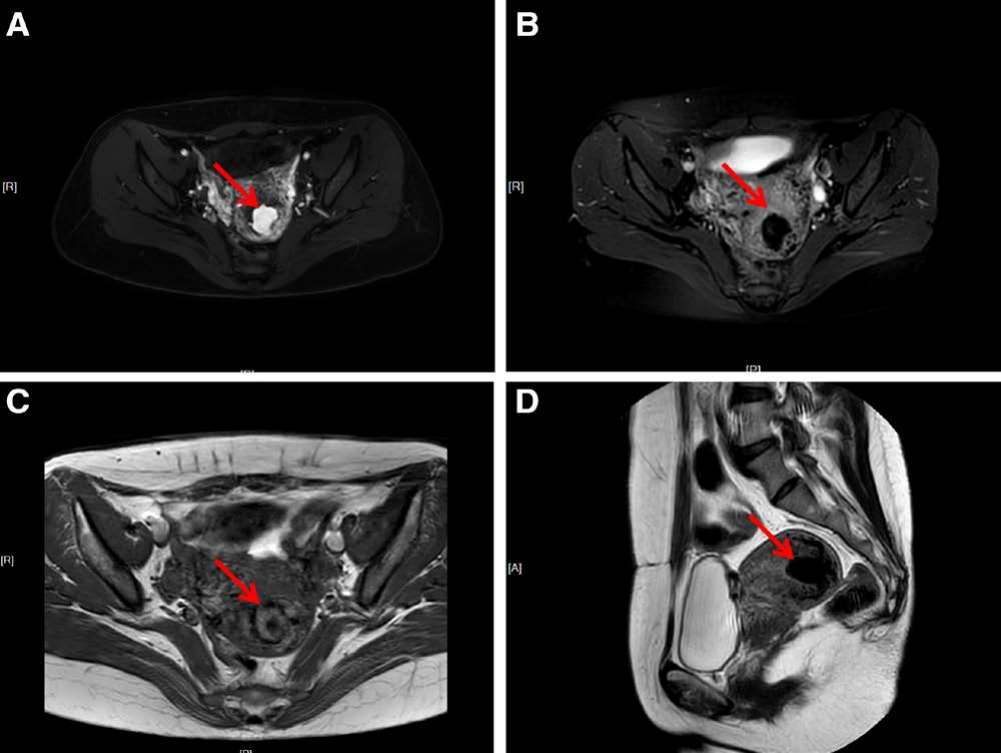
Pelvic MRI findings suggestive of an abnormal intrauterine vascular mass with possible aneurysmal formation (arrow). (A) Axial T1WI-FS image. (B) Axial T2WI-FS image. (C) Axial T1WI image. (D) Sagittal T2WI image. MRI = magnetic resonance imaging.
